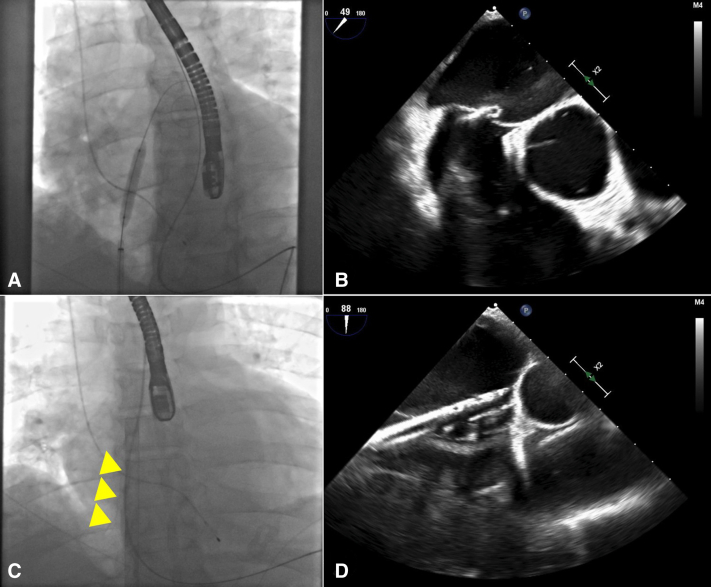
Steps for left atrial venous cannula placement. Femoral venous access is obtained and an 8-French Baylis transseptal system is used with TEE guidance for transeptal access. A, A wire is placed in the left upper pulmonary vein and an atrial septostomy done using an 8- × 40-mm balloon. B, TEE showing transseptal access and balloon inflation. C, 25-French ECMO cannula with multiple fenestrations at the distal end is advanced. D, TEE showing the distal end of the cannula positioned 3 cm across the interatrial septum for drainage from both atria. *TEE*, Transesophageal echocardiography; *ECMO*, extracorporeal membrane oxygenation.
